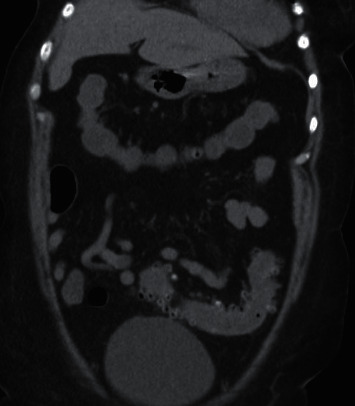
CT abdomen and pelvis without contrast, showing mild inflammation surrounding the sigmoid colon. Diverticulosis is present.
